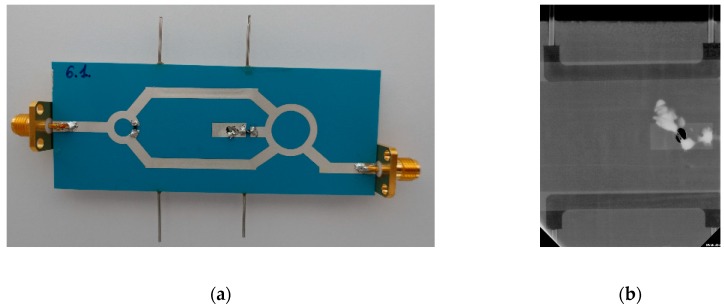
The coupler-based sensor: (a) photo, (b) X-ray image of microstrip lines and channel alignment.
